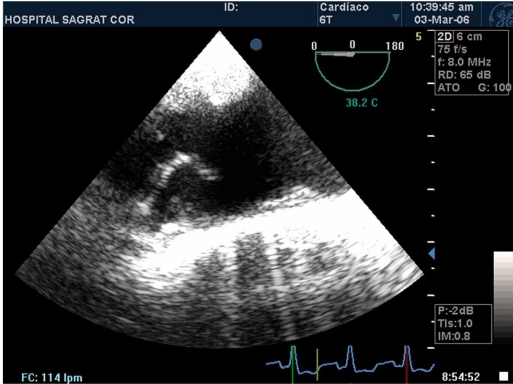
Transesophageal echocardiogram showing an aortic arch complex plaque with a mobile component.
